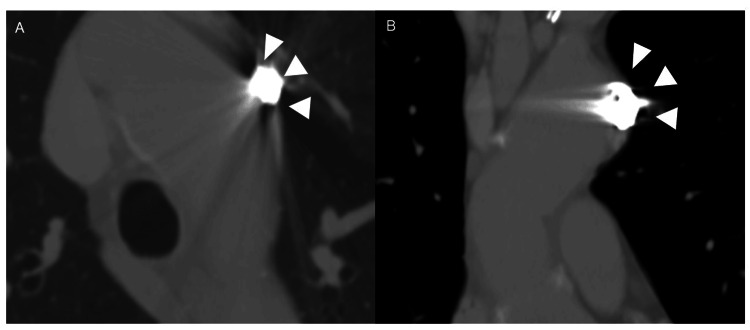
The CT findings three years after coil embolization of the ULP The CT findings three years after coil embolization of the ULP (A, B) show that the ULP had almost disappeared, with no residual blood flow (white arrowheads). ULP: ulcer-like projection; CT: computed tomography
